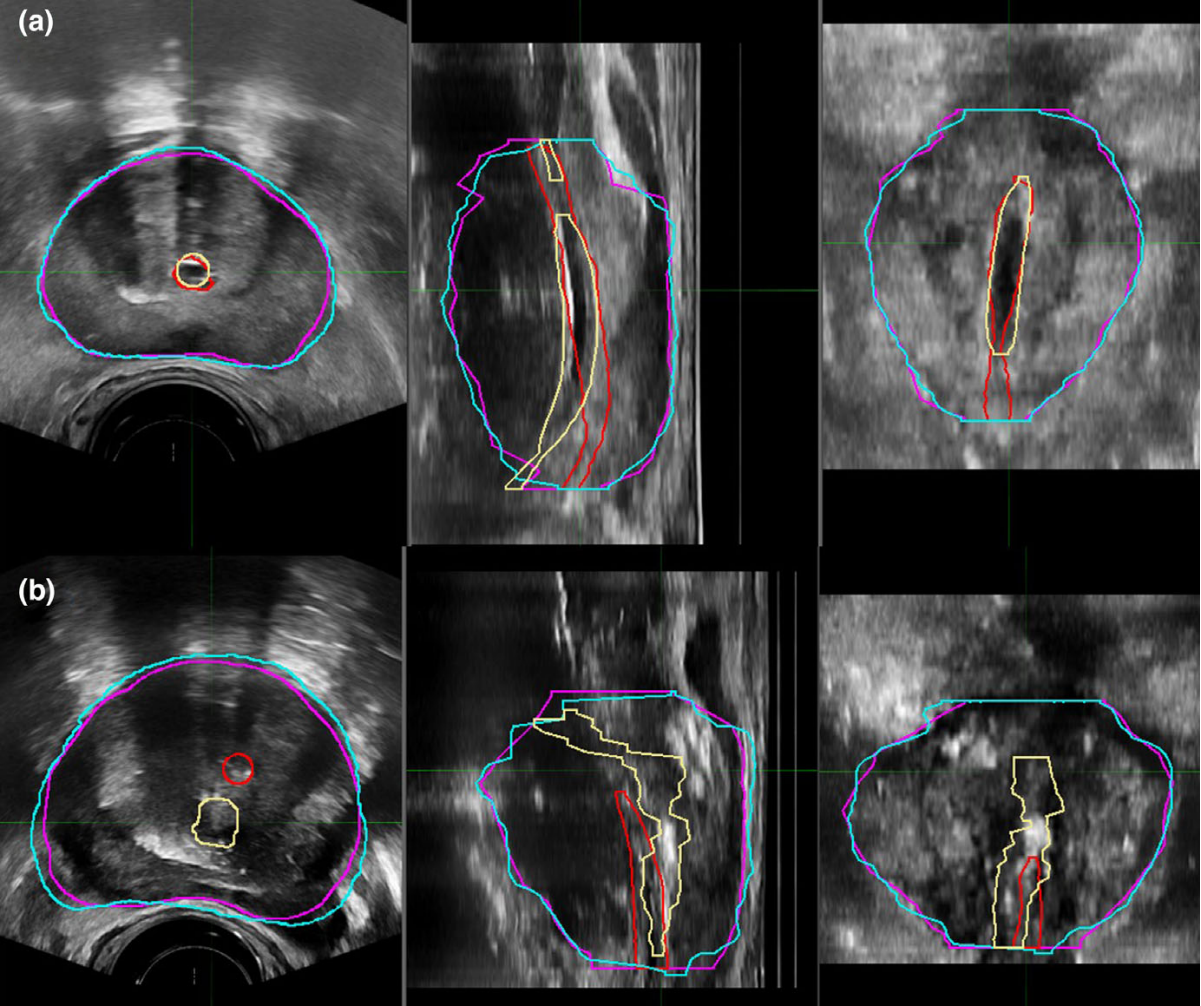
Example of a good (a) and a poor (b) contour‐based deformable image registration. In both panels, the TRUS contours for the prostate and urethra are shown in purple and red, respectively. The deformed MRI contours for the prostate and urethra are shown in cyan and yellow, respectively.
